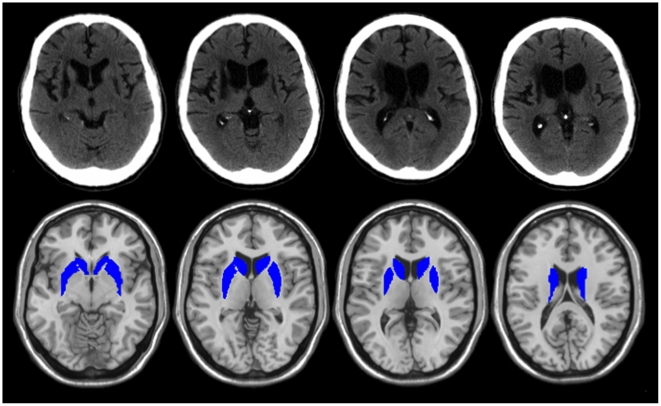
CT images from KQ-167 demonstrating loss of the caudates bilaterally (with ventricular enlargement) and infarcts in the right and left lenticular nuclei. Overlay images of the intact basal ganglia on a template brain are displayed for reference purposes.
